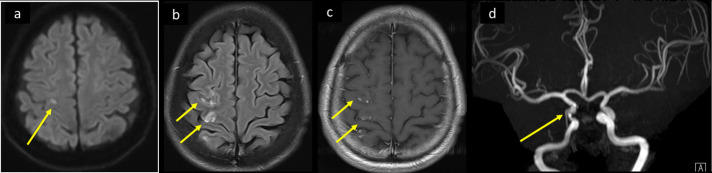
A 47-year-old male who was a chronic smoker, and had DM, HTN presented to the ED, with history of two episodes of weakness of the left side of the body that lasted for 1 h and totally resolved. He had fever and mild respiratory symptoms and tested positive for COVID-19. Axial MRI brain images at supraventricular level, (a) DWI b 1000, (b) T2-FLAIR and (c) *T*_1_WI post i.v. contrast show precentral tiny lacunar diffusion restriction with bright signal on DWI-b 1000 (arrow in a), pre- and post-central cortical and subcortical areas of bright signal on FLAIR *T*_2_WI, and gyral post-contrast enhancement (arrows in b, c respectively) consistent with acute to evolving subacute ischemic changes. This correlates with the resolved episodes of TIAs. (d) Intracranial MIP- 3D-MRA shows significant narrowing with mural irregularity of cavernous segment of right ICA (arrow in d) which probably along with COVID-19 increased thrombogenicity led to more distal small vessel emboli. DM, diabetes mellitus; ED,emergency department; FLAIR, fluid attenuated inversion recovery; HTN, hypertension;ICA, internal carotid artery; MIP, maximum intensity projection; MRA, MRangiography; TIA, transient ischemic attack.
